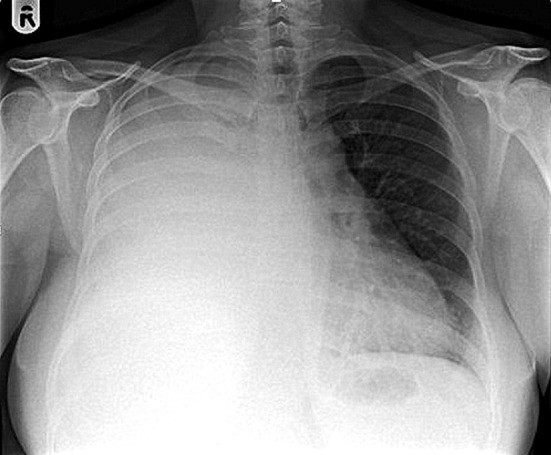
Chest radiograph demonstrating complete opacification of the right hemithorax with mild mediastinal shift to the left side.
